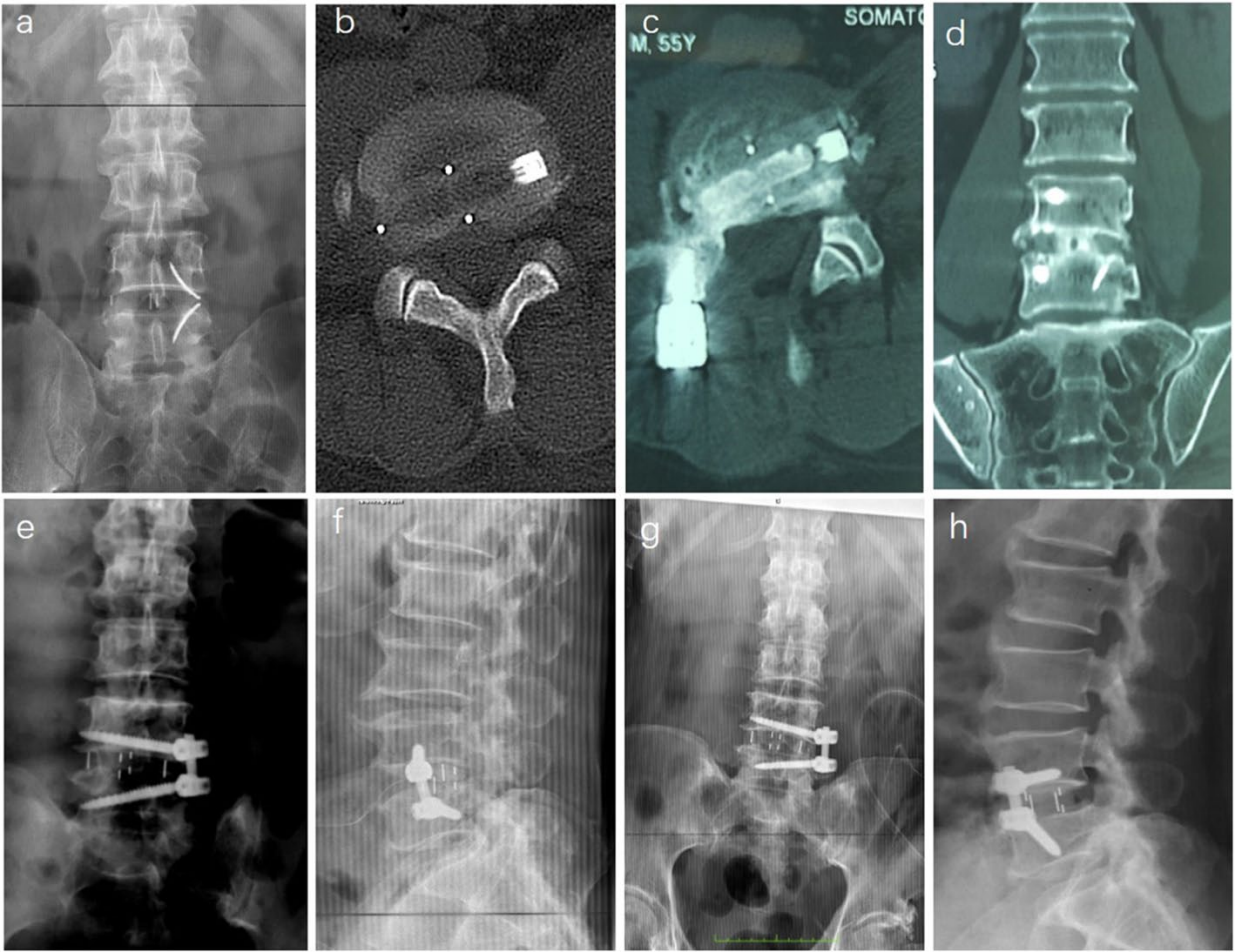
A case of right nerve root compression using TLIF revision a-d. a Anterior X-ray after XLIF. b Axial CT showed that the right nerve root was compressed by cage. c, d Axial and coronal CT showed direct decompression of nerves and fusion one year after right TLIF. A case of revision in which the position of the screw was adjusted through the original incision e-h. e, f Anterior and lateral X-ray after XLIF. g, h Anterior and lateral X-ray showed the position of screw was adjusted through the original incision, and the symptoms were relieved
